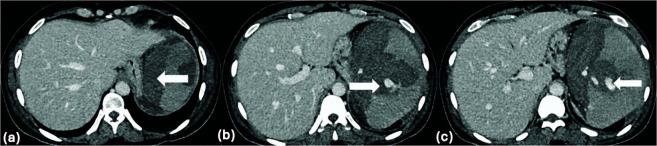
Axial images of the CT scan of the abdomen with IV contrast in venous phase: (a) subcapsular hematoma (white arrow); (b) and (c) multiple foci of active IV contrast extravasation (white arrow), suggestive of active bleeding, in addition to the splenic laceration and hematoma.
